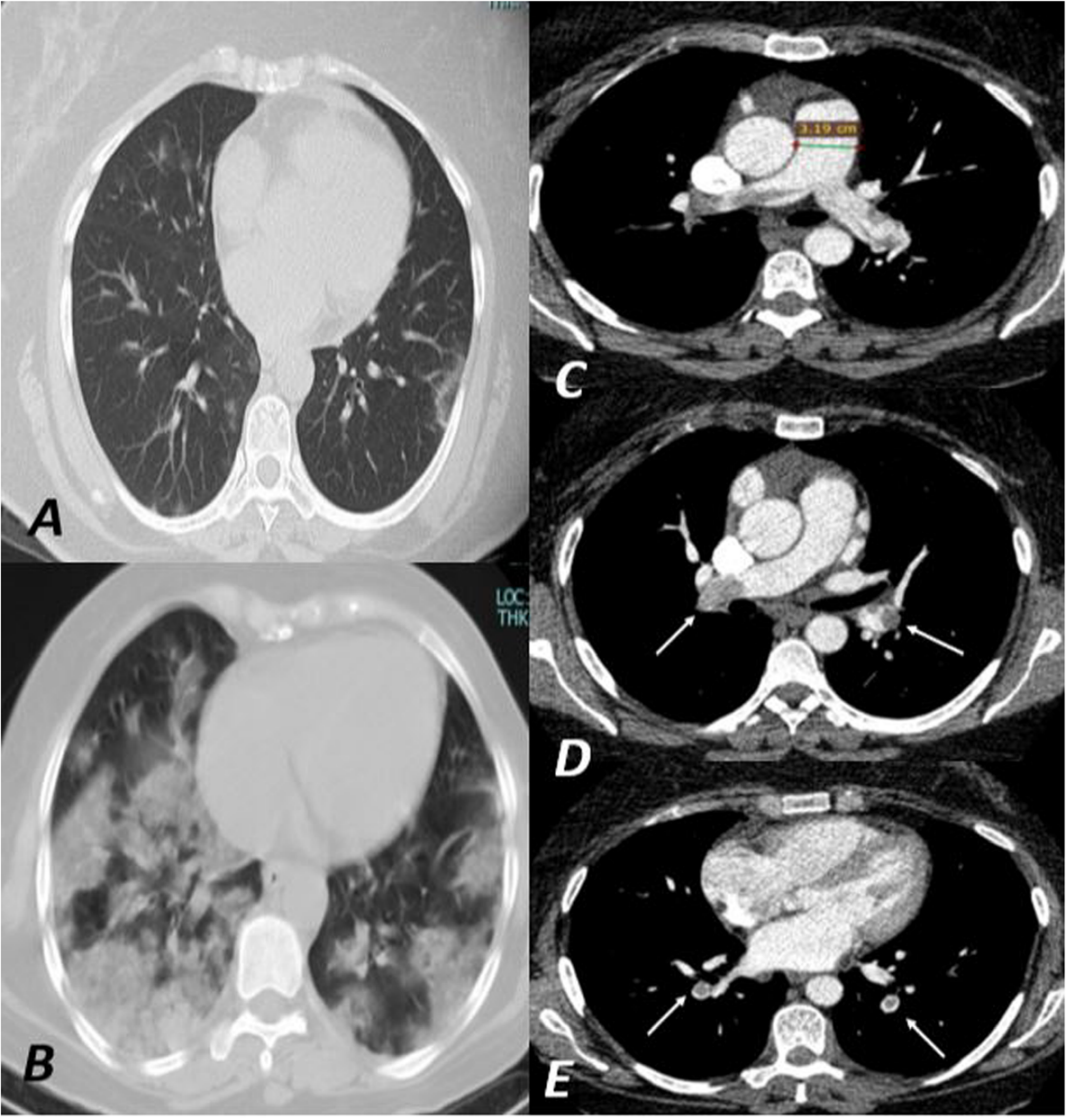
A female patient 48 years old known case of COVID-19 infection as confirmed by PCR. She complained of chest pain 12 days after the onset of the disease and her oxygen saturation level showed a gradual decrease. Her D-dimer level was 1.1 μg/ml and repeated to show a gradual increase reaching 1.8 μg/ml. a The axial CT image of the baseline study of the patient showing a few bilateral peripheral patchy areas of ground-glass opacity while b was her follow-up after a recent complaint showing a progressive course of the disease extension. A pulmonary embolism was suspected for which CTPA was done and revealed a saddle pulmonary embolism involving the right and left main pulmonary arteries extending into the lobar branches (white arrow in image d and e). Positive RV strain was identified with dilated main pulmonary artery (31.9 mm in image c), reversed RV/LV ratio, and leftward deviation of the IV septum (image e)
